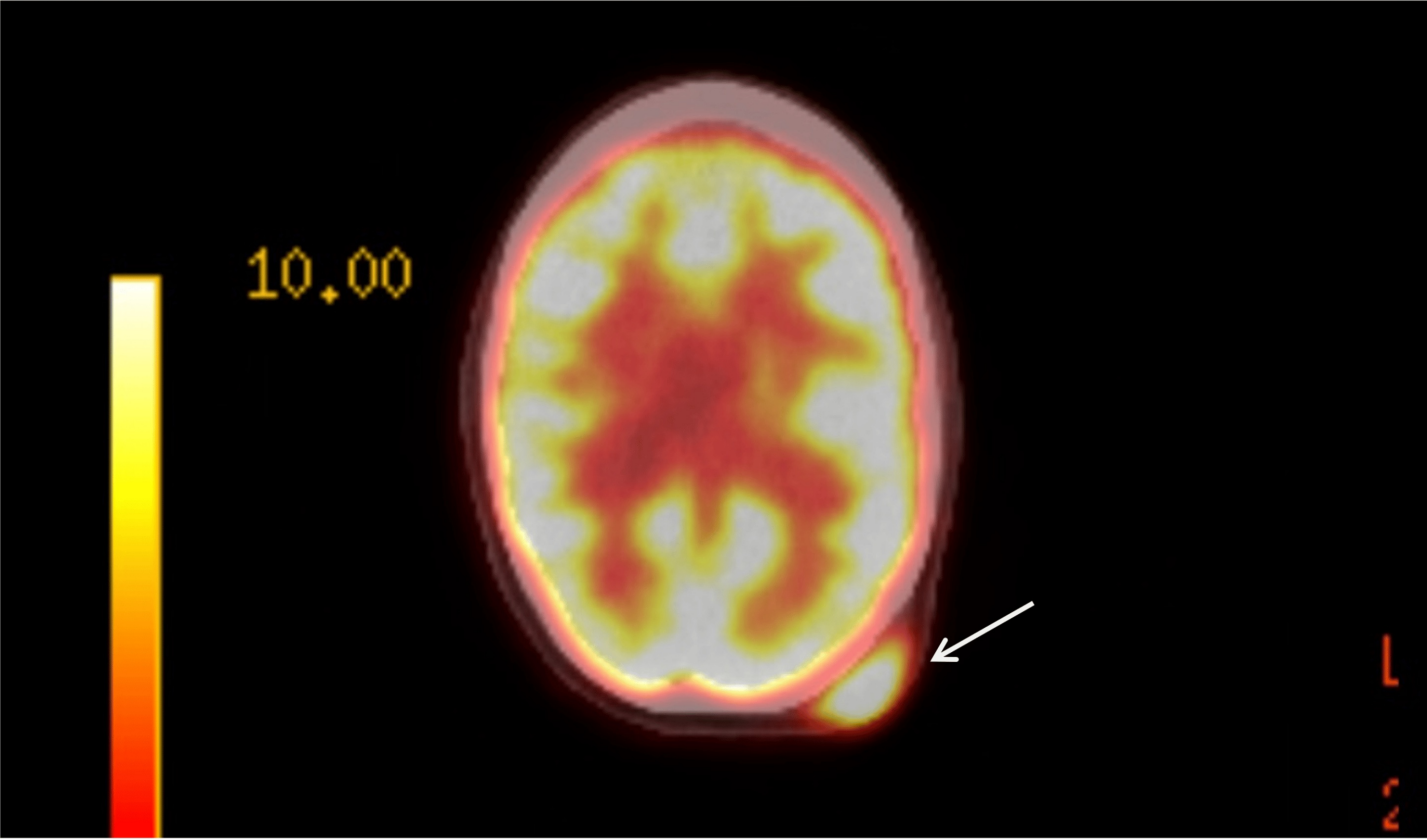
PET demonstrating FDG avid uptake in the arrow of known mass PET: positron emission tomography; FDG: fluorodeoxyglucose
